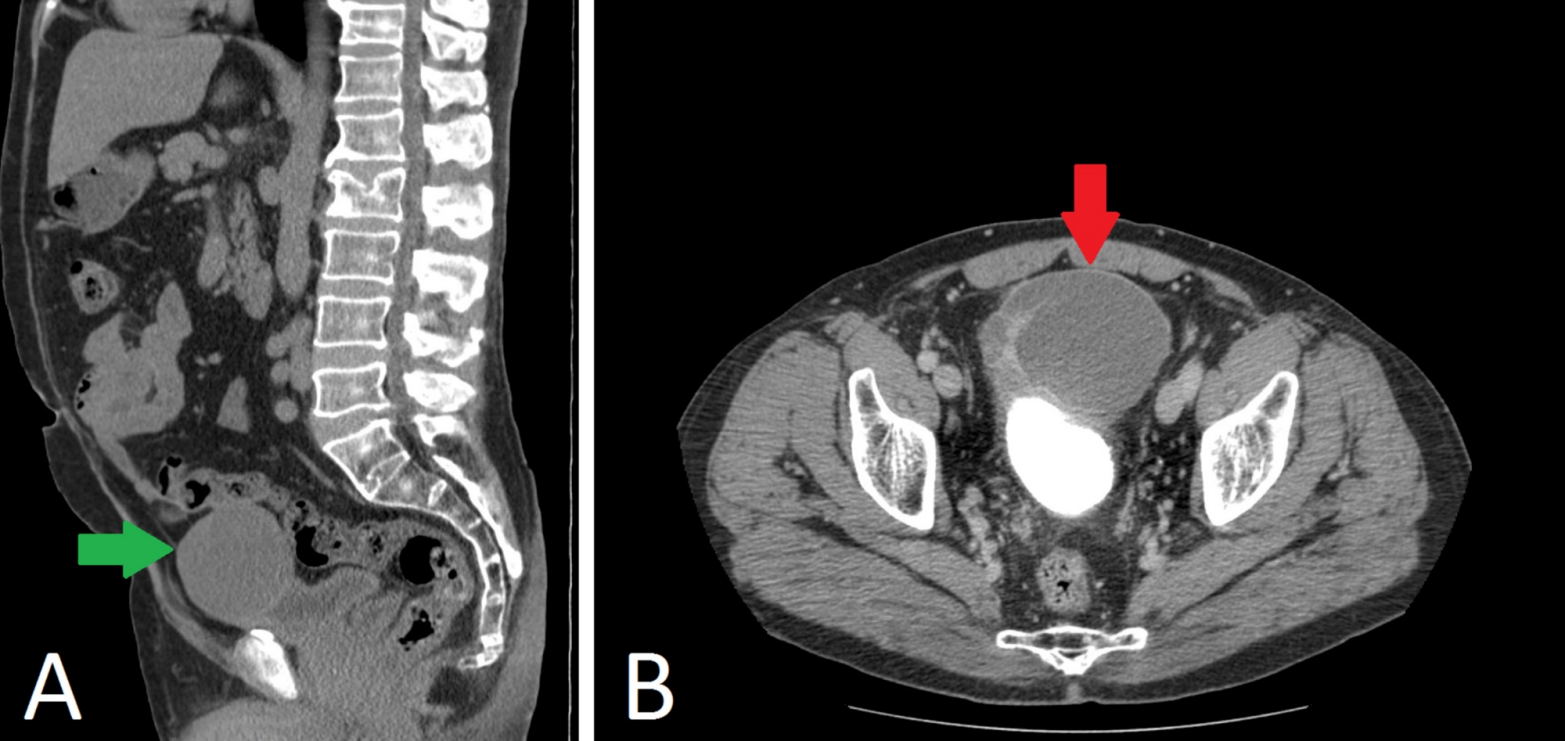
Contrast-enhanced CT scan of the abdomen and pelvis demonstrating the multiseptated cystic mass measuring 6.4 x 7.3 x 7.0 cm in anteroposterior, craniocaudal, and transverse dimensions, which contains multiple thickened, enhancing septations and eccentric wall thickening. This mass is abutting, but not infiltrating the adjacent large bowel wall, and there is a clear fat plane between the anterior aspect of the mass and the inner surface of the lower central abdominal wall. (A) Sagittal plane (green arrow). (B) Axial plane (red arrow).
